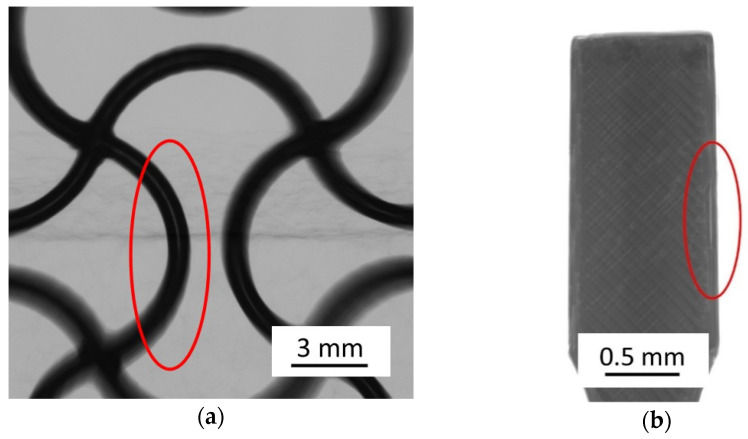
(a) Radiography of auxetic green body with adhesion defects between two outlines, (b) Radiography of tension rod with adhesion defects in infill +45°/−45°and between infill and outline.
